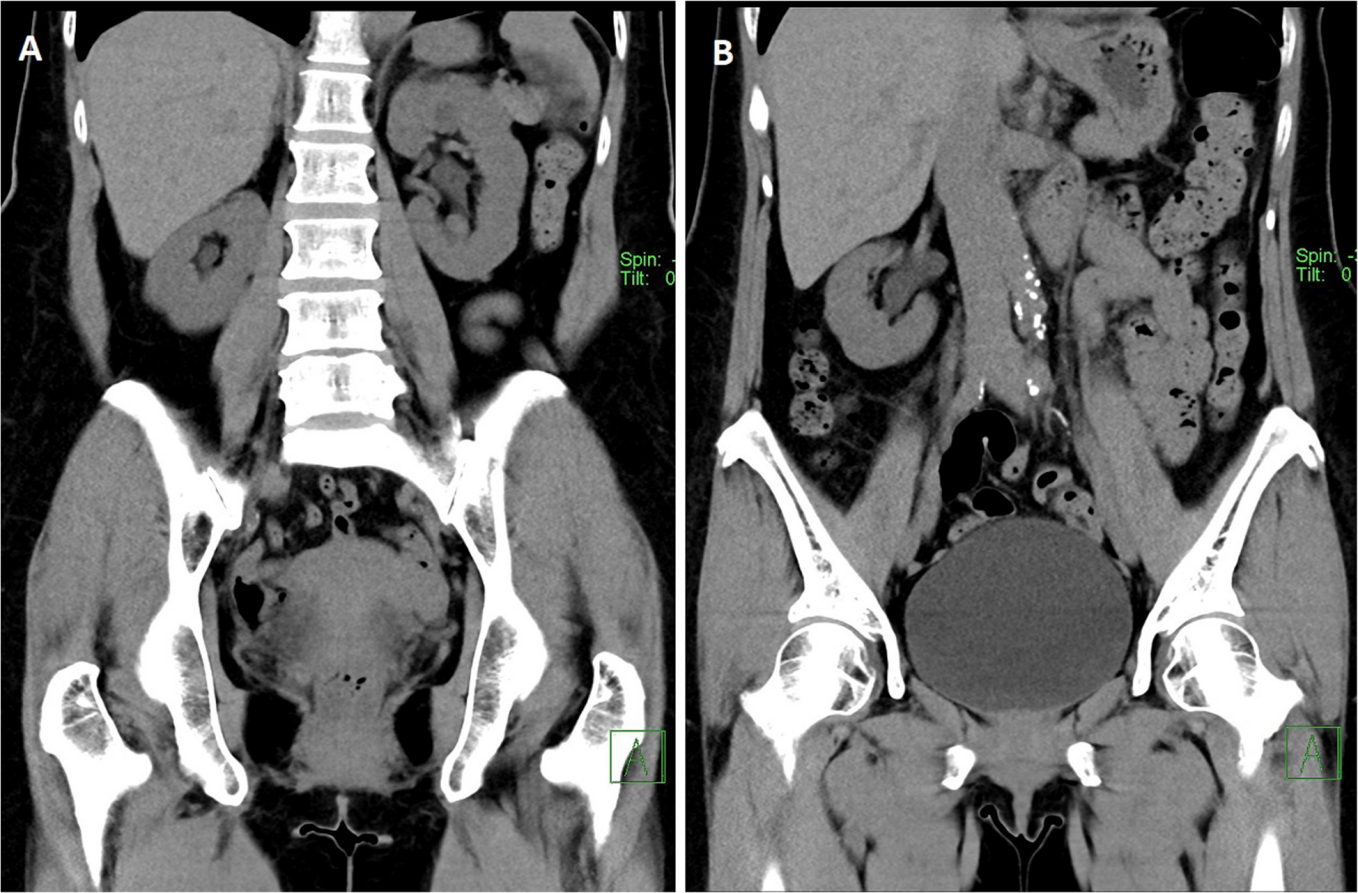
Non-contrast phase of the CT urogram showing no hydronephrosis or hydroureter bilaterally (a), and a well-distended urinary bladder (b). No ureteric or bladder calculus was seen
